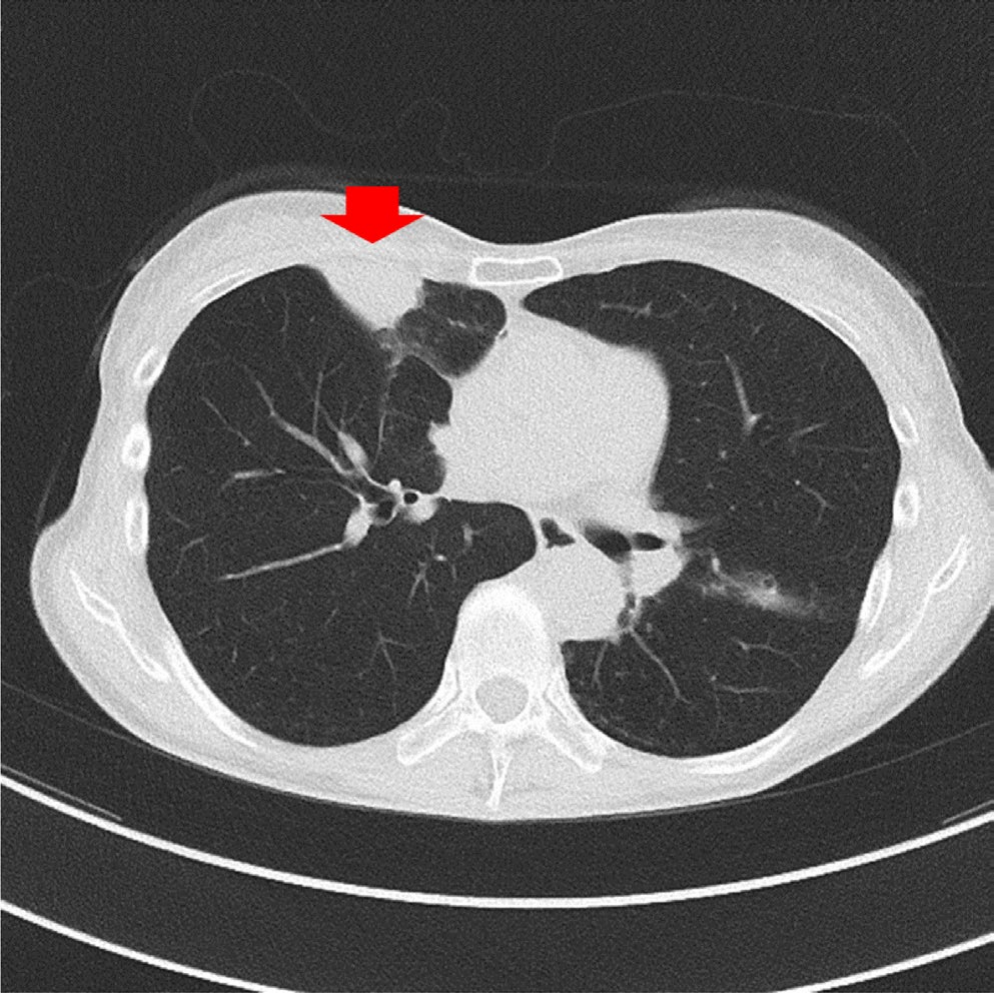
Chest computed tomography (CT) scan of a 68‐y‐old female with postoperative left upper lobe lung cancer. A large lesion is seen in the right middle lobe, which was diagnosed as *Mycobacterium avium* using sputum that was collected by endotracheal suctioning
